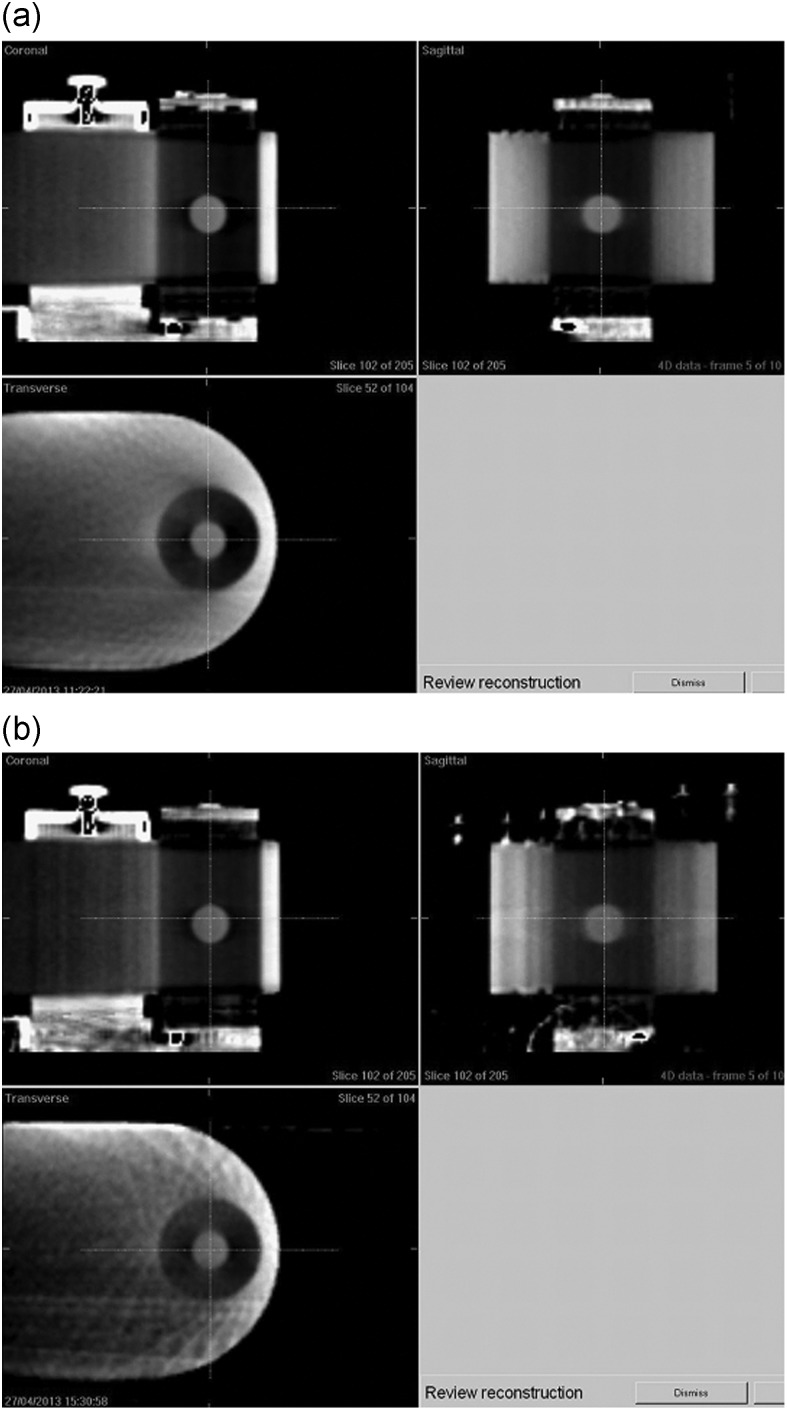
A comparison of inhalation-phase images of concurrent 4D CBCT during VMAT delivery with (a) FF and (b) FFF.
